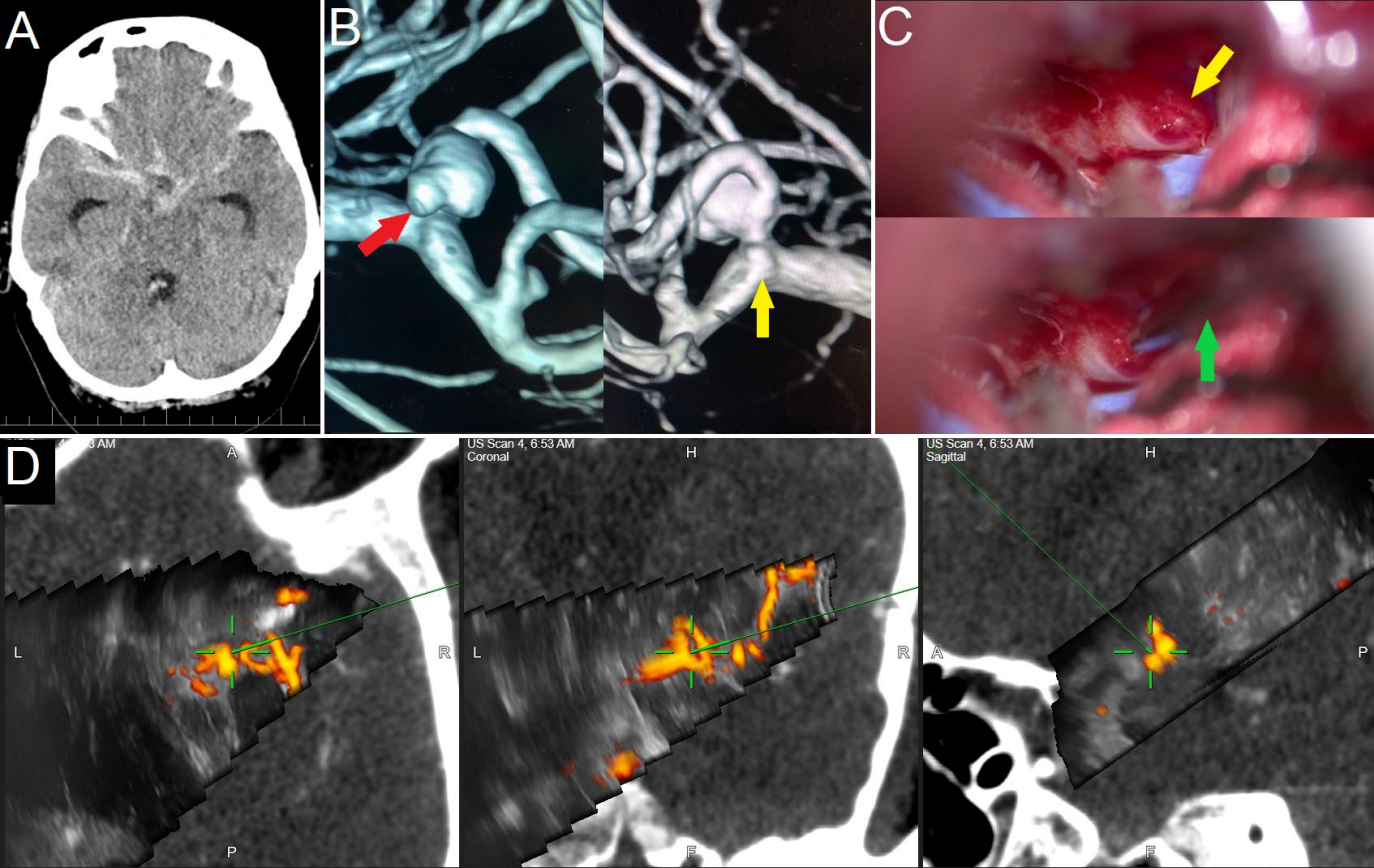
Ruptured right-sided MCA aneurysm – case No. 25. A: Preoperative CT showing massive subarachnoid hemorrhage (modified Fisher scale grade IV.). B: 3D reconstructions of rotational DSA, presumed site of the rupture was the aneurysm daughter sac *(red arrow)*. In addition, a bleb in the aneurysm neck was depicted as well *(yellow arrow)*. Due to the aneurysm morphology the endovascular treatment was refused by interventional radiologists. C: Intraoperative photographs. *Upper image: *A small extremely thin-walled blister aneurysm *(yellow arrow)* was found during Sylvian fissure dissection. *Lower image:* Navigation pointer (green arrow) was used for better anatomical orientation. D: Updated 3D-US power-Doppler scan confirming the location of the blister aneurysm is in the MCA bifurcation. Subsequent manipulation with MCA was considered to be unacceptably dangerous; the patient was subsequently treated endovascularly on a delayed basis after 2 weeks of controlled normotension
